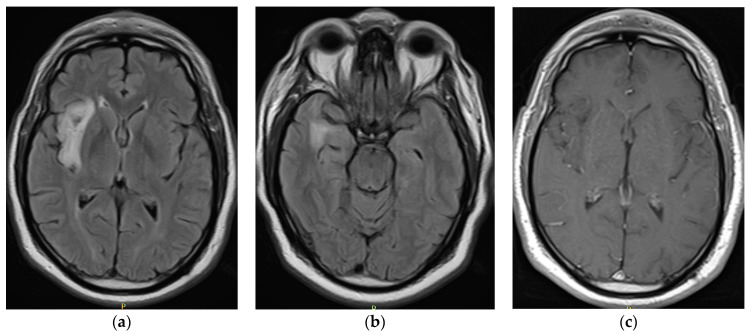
September 2021: iRM FLAIR axial (a,b) and T1 gadolinium (c) images.
